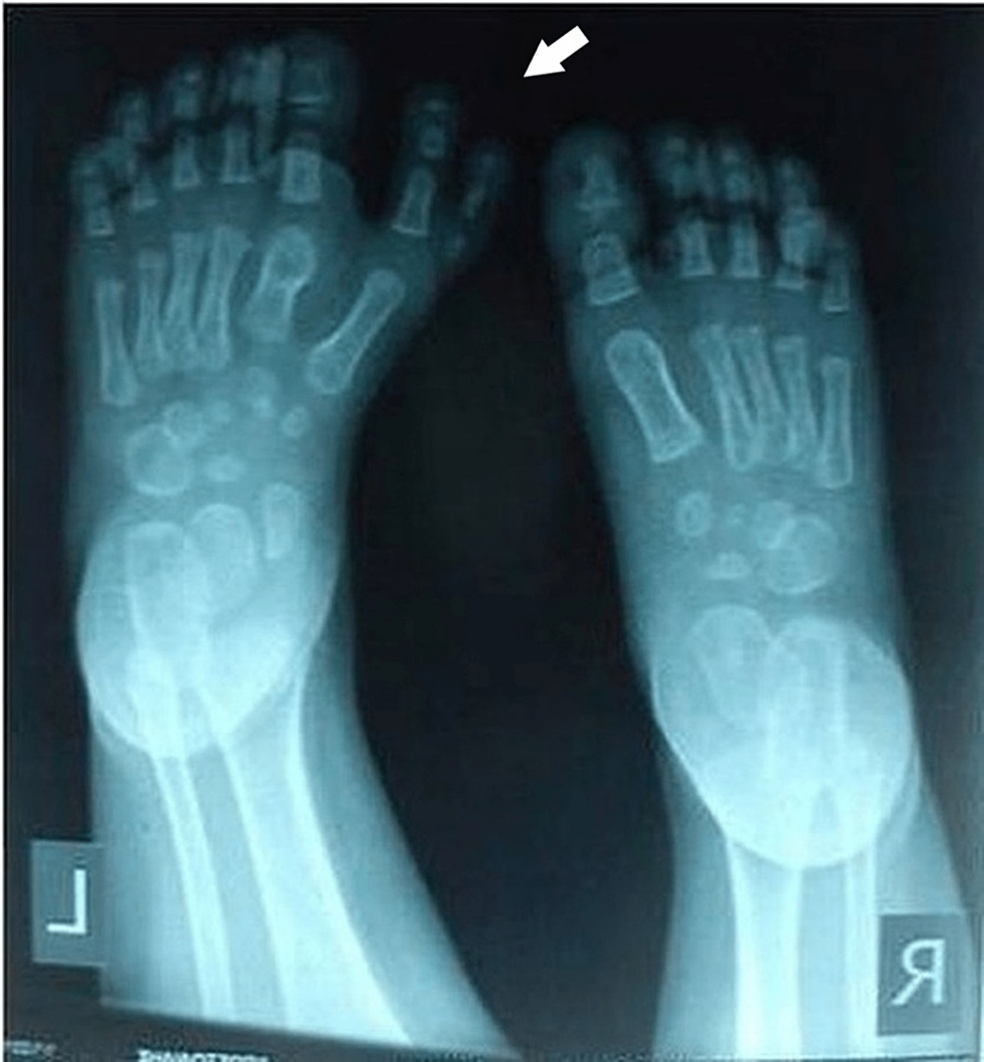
X-ray of the first patient's affected foot. X-ray with duplication of the medial rays, with both supernumerary toes having three phalanges, with hypoplastic the medial part. There were six metatarsals, supernumerary cuneiforms, and hypoplastic supernumerary navicular. The second metatarsal, corresponding to the normal great toe, had a proximal position of the epiphysis.
